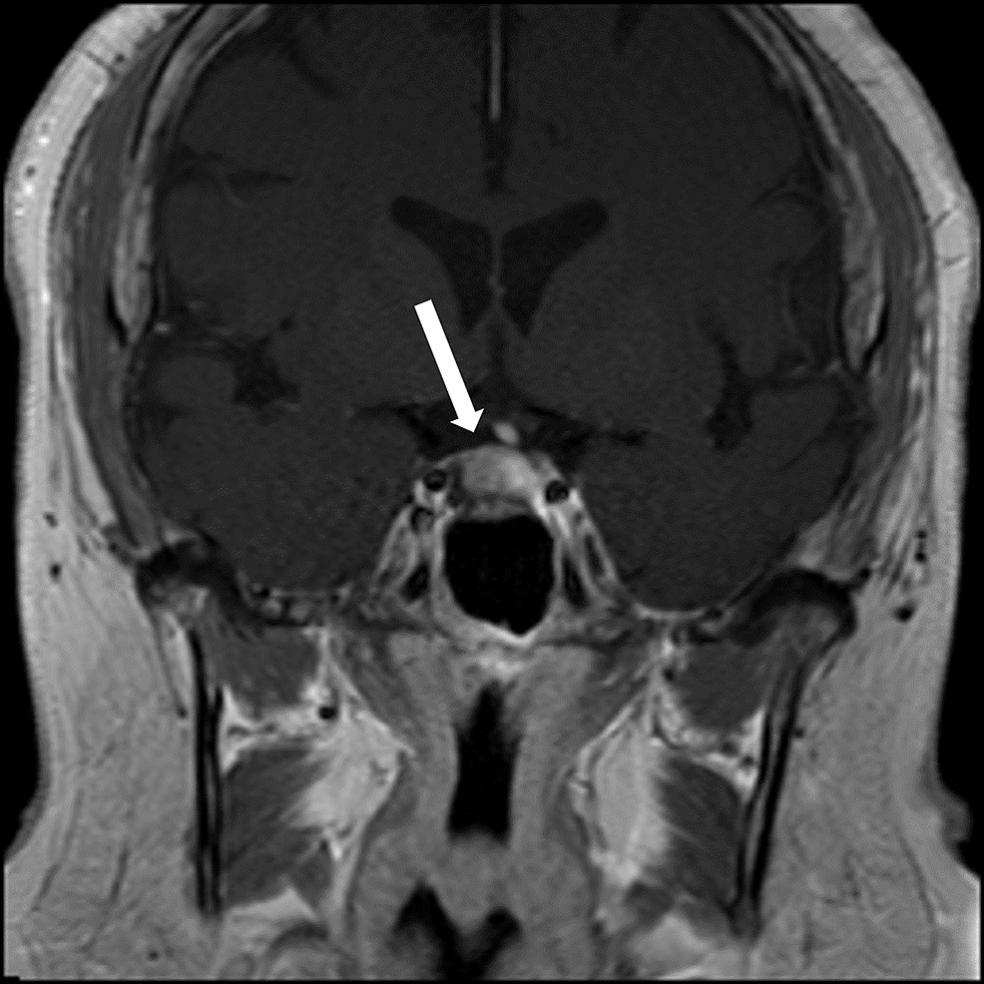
T1-weighted coronal MRI of the pituitary demonstrating a 1.1 cm × 1.5 cm × 1.1 cm cystic sellar mass which represents a pituitary macroadenoma (arrow).
